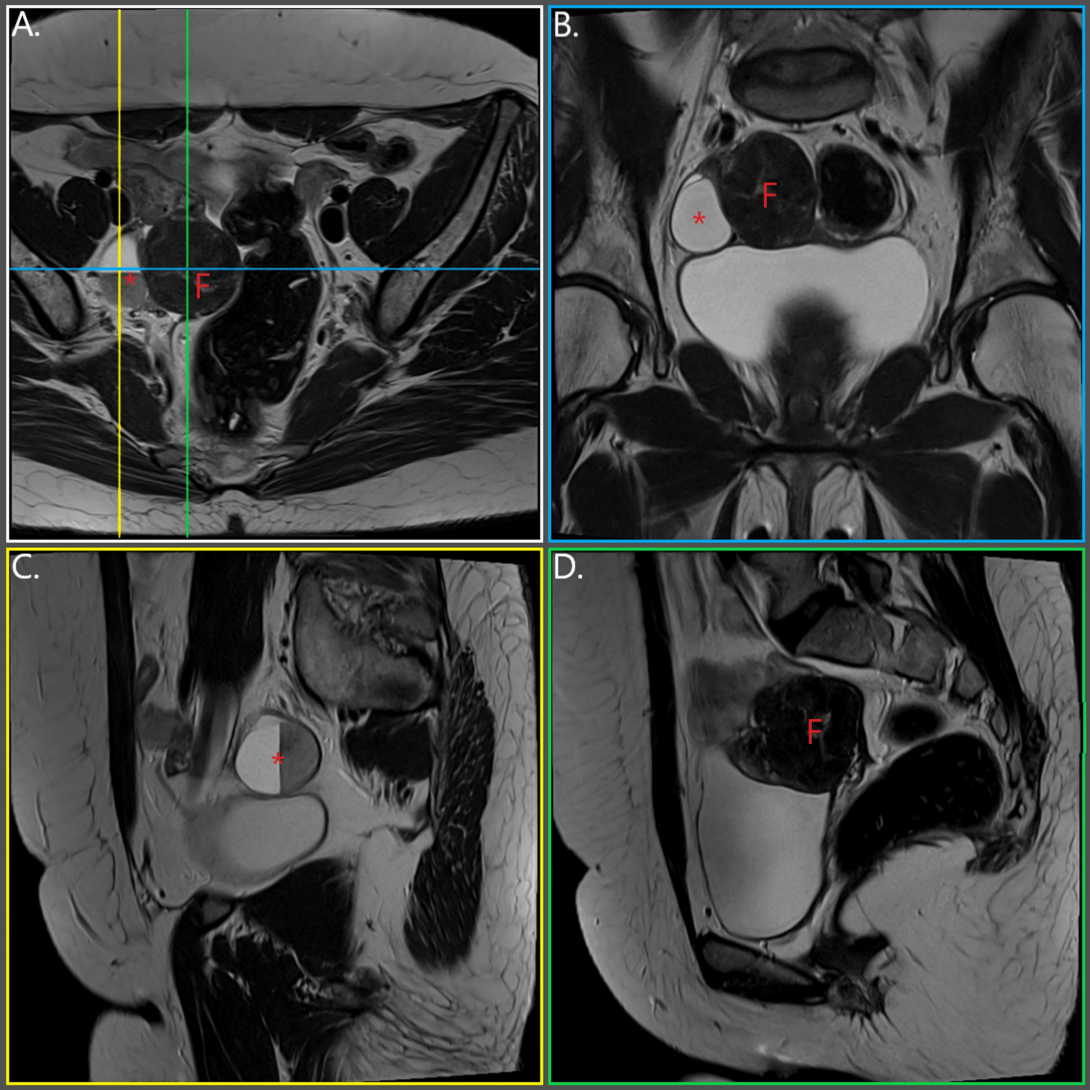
Preoperative pelvic and lower abdomen MRI scan, multiplanar reconstruction F: uterine fibroid; *: ovarian cyst (A) transverse plane; (B) coronal plane (blue); (C) longitudinal plane, level of ovarian cyst (yellow); (D) longitudinal plane, level of fibroid
